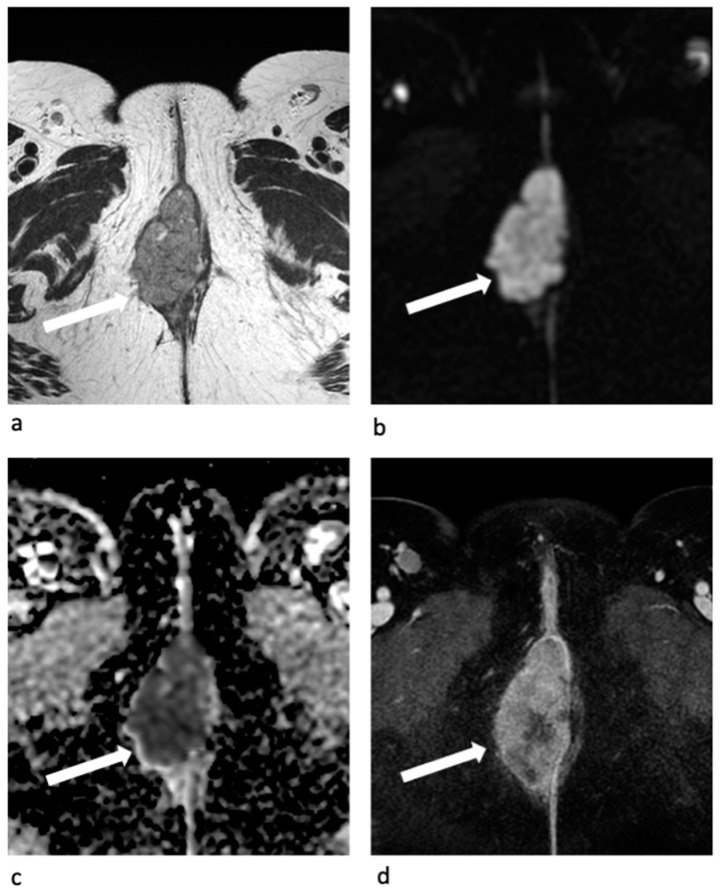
MR images of vulvar tumor. The tumor (arrow) shows intermediate hyperintense signal intensity on axial T2-W MR image («evil grey») (a) and hyperintense signal intensity on DW-MR image (b) with restricted diffusion on the corresponding ADC map (c) due to the high cellularity. On the axial contrast-enhanced T1-W MR image (d) acquired during the arterial phase the tumor shows early and conspicuous enhancement.
